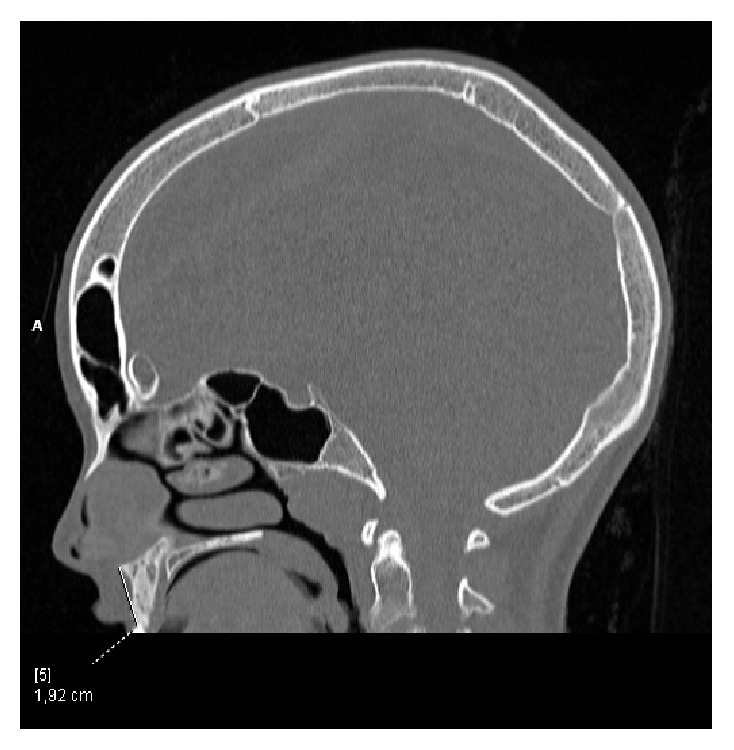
An example of ns/pr distance marked on the CT image.
